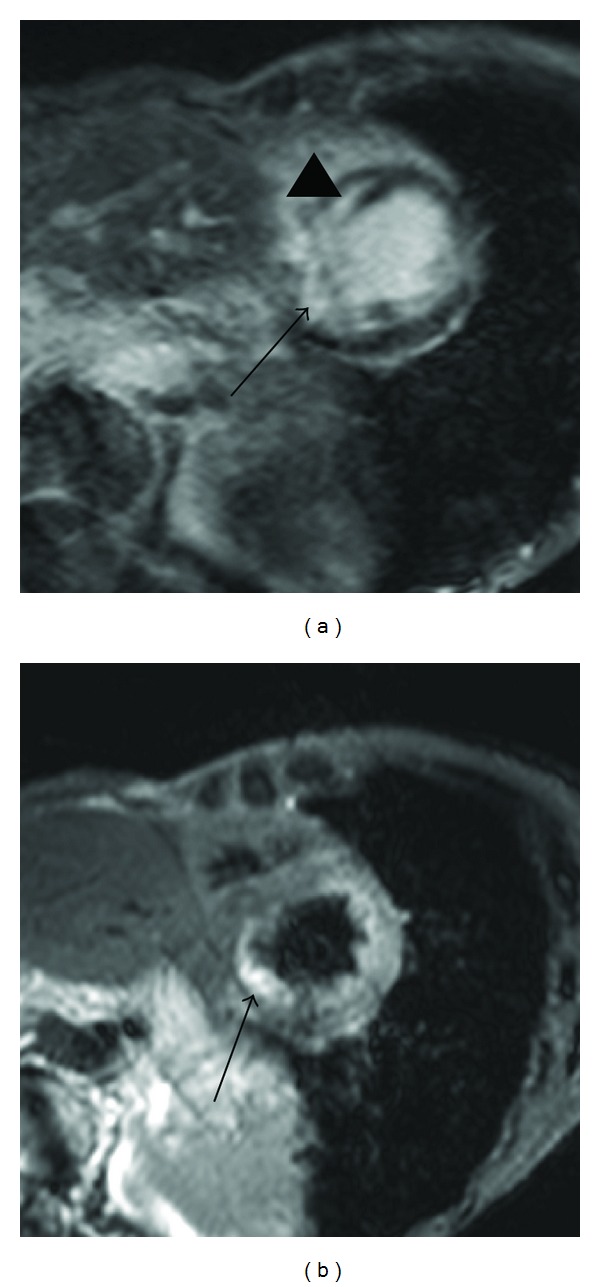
73-year-old female with cardiac sarcoidosis presenting with lower ejection fraction and conduction disturbance. Short-axis delayed-enhancement MRI (a) shows abnormal enhancement consistent with the edema (arrow) as well as the septal mesocardial myocardial scarring (arrowhead). T2-weighted cardiac MRI (b) shows myocardial edema at the transmural myocardium of the inferior region (arrow).
